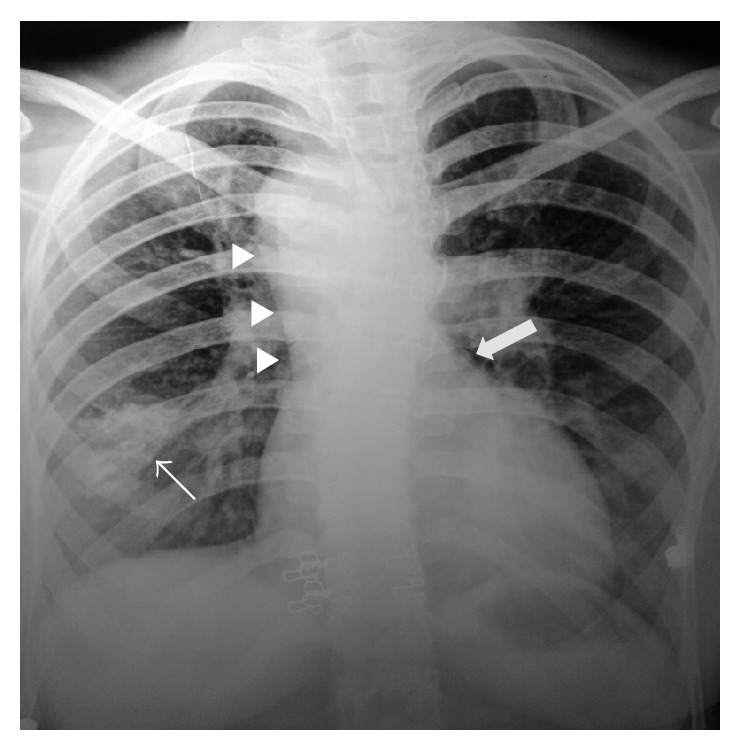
Chest radiograph: frontal view reveals left hilar prominence, right sided aortic arch (arrowheads), absent pulmonary conus (thick arrow), and a small ill-defined calcified nodular radio-opacity in the right lower zone of the lung (thin arrow).
